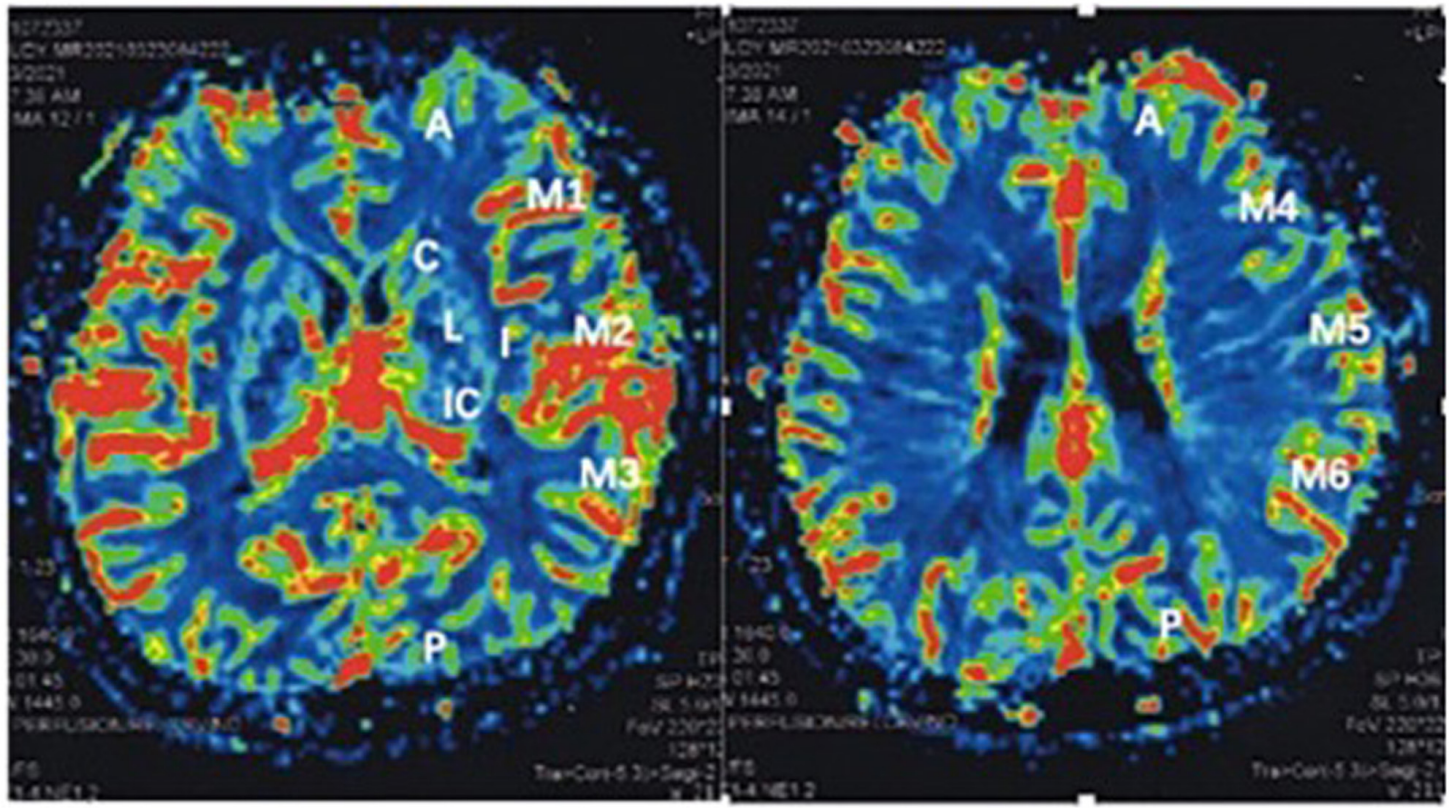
According to the ASPECTS study [9], the MCA supply area is at two standardized regions (basal ganglia and supra-ganglionic levels). The standardized areas are divided into ten sections: C, L, IC, I, M1, M2, and aM3 at the level of basal ganglia area and M4, M5 and, M6 at the level of the supra-ganglionic area. Legend: A, the anterior cerebral artery supplied areas; P, the posterior cerebral artery supplied areas; C, the areas of the caudate nucleus; L, the areas of the lenticular nucleus; IC, the areas of the internal capsule; I, the areas of the insular zone. M1–M6 represent the MCA supplied areas.
